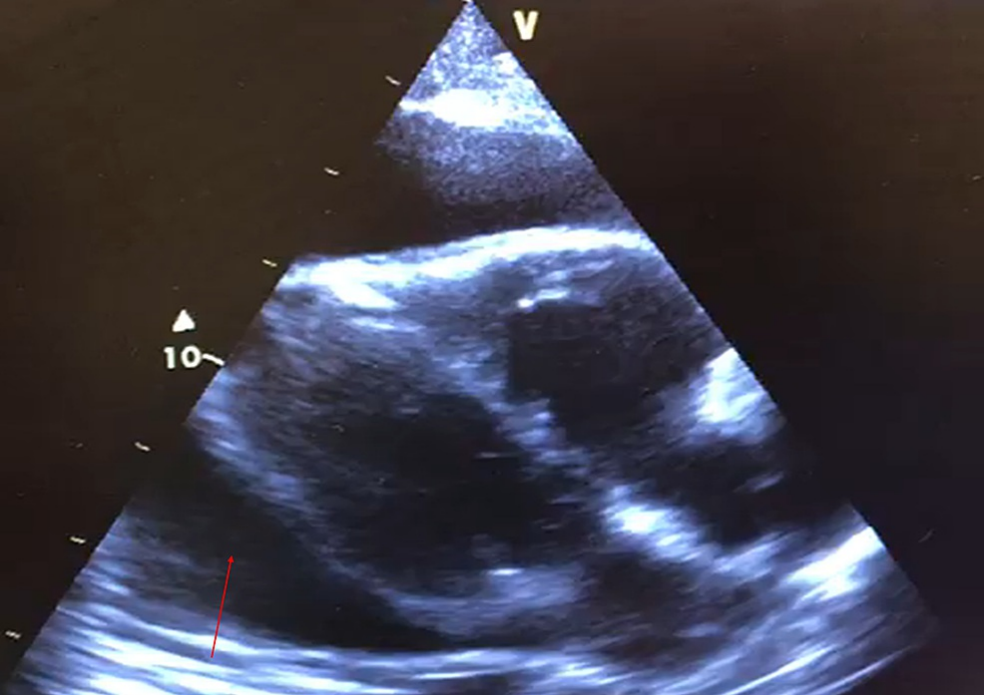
Transthoracic echocardiography. Intrinsically normal heart and a large pericardial effusion (arrow).
